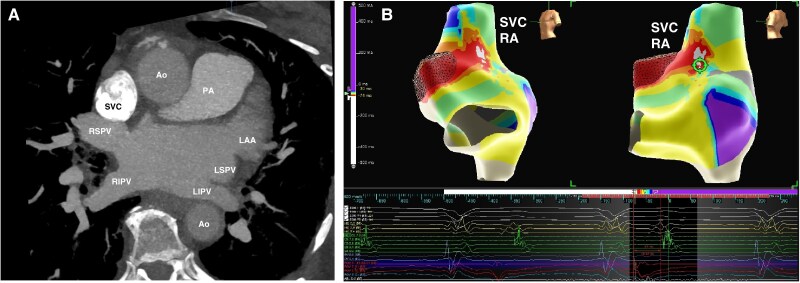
(*A*) Transverse axis view of cardiac CT shows contrast filled superior vena cava that is situated adjacently anterior and superior to the right superior pulmonary vein. Other labelled structures are the right inferior pulmonary vein (RIPV) on the right, the left superior pulmonary vein, the left inferior pulmonary vein, and the left atrial appendage on the left, aorta and pulmonary artery anteriorly, and descending aorta posterior to the left inferior pulmonary vein. (*B*) Electroanatomic (Ensite NavX, Abbott Cardiovascular, USA) local activation map of atrial tachycardia (cycle length 350 ms), colour coded for early to late activation sites. Atrial tachycardia confined to the superior vena cava-right atrial junction was ablated terminating the tachycardia and isolating superior vena cava. Ao, aorta; CT, computed tomography; LAA, left atrial appendage; LIPV, left inferior pulmonary vein; LSPV, left superior pulmonary vein; PA, pulmonary artery; RA, right atrial; RIPV, right inferior pulmonary vein; RSPV, right superior pulmonary vein; SVC, superior vena cava.
